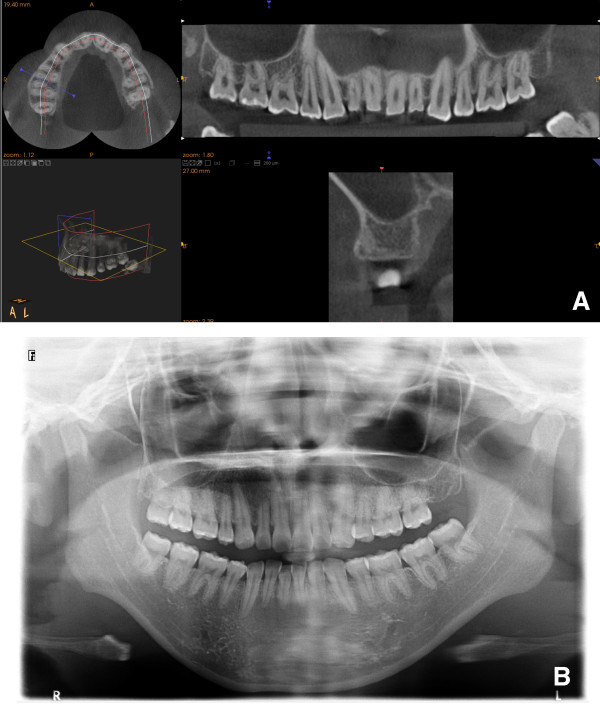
Typical images of CBCT (A) and panoramic radiographs (B) in the present study. The CBCT can visualize the area up to 35 mm above the patient’s occlusal plane and between the bilateral maxillary retromolar positions.
